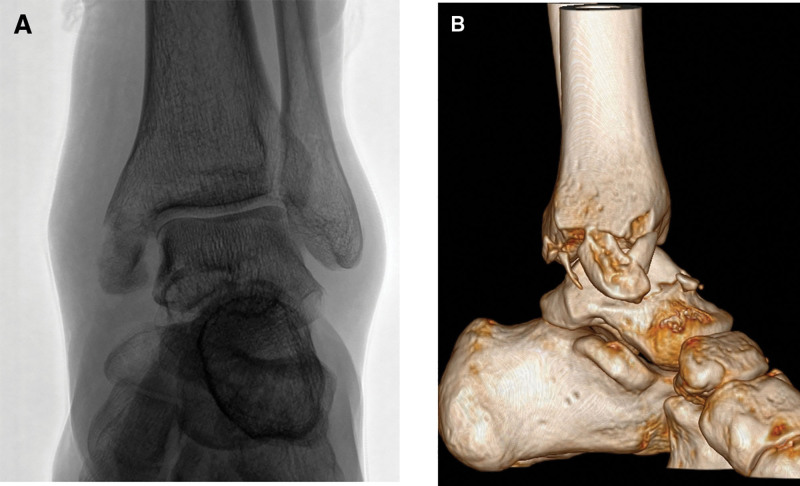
Preoperative imaging of the fractures. (A) X-ray showing a comminuted medial malleolar fracture. (B) Computed tomography scan revealing the comminuted medial malleolus fracture (including anterior and posterior fragments) and the talar neck fracture.
